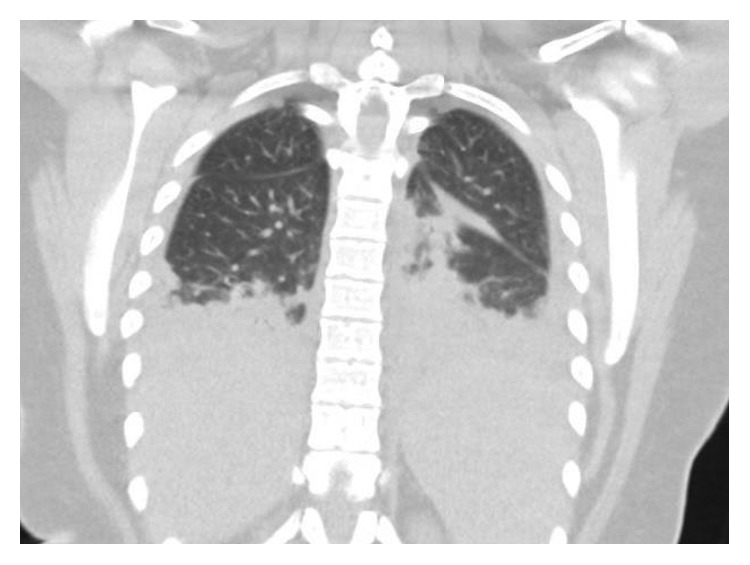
Computed tomography (CT) chest without contrast showed bilateral lung nodules and pleural effusions.
